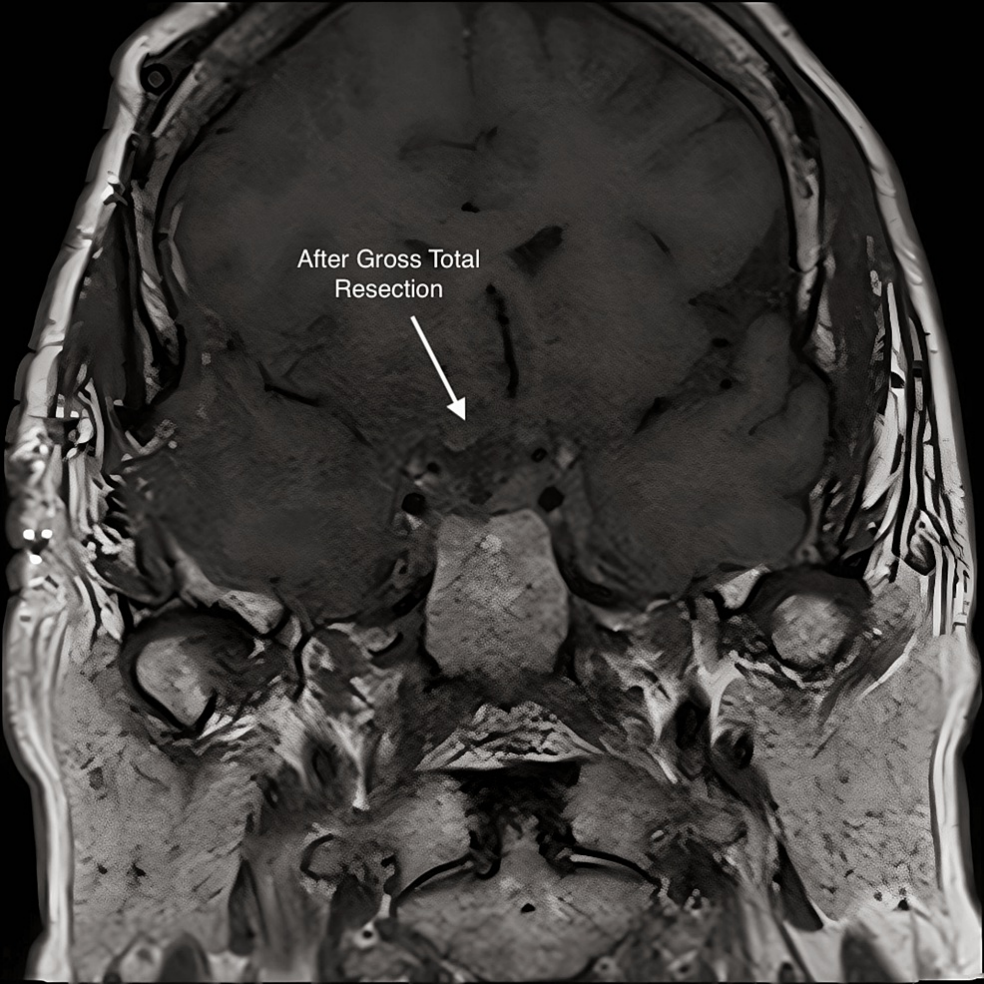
Mid-coronal MRI with contrast; this was taken after subfrontal craniotomy with gross total resection resulting in resolution of headaches and “brighter” vision
